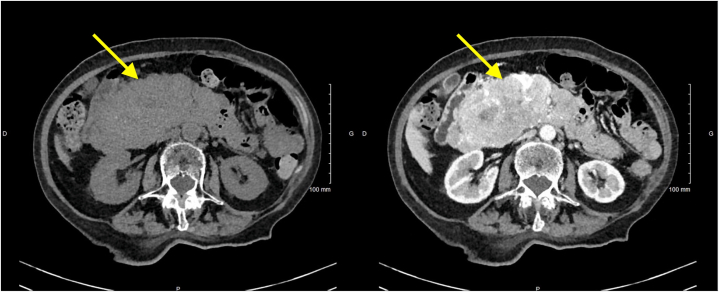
Contrast-enhanced abdominal CT scan showing a large, heterogeneous mass measuring 120 × 150 × 64 mm located in the pancreatic head. Arrows indicates the pancreatic mass. *CT*, Computed tomography.
